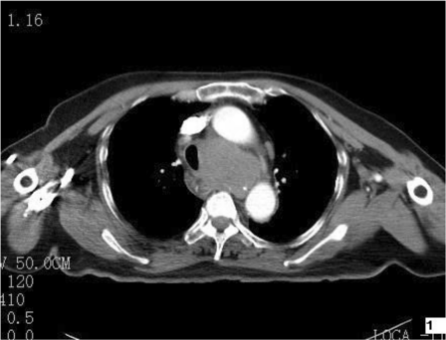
Axial contrast-enhanced CT image of the chest shows a well-defined lobulated homogeneous soft tissue mass in the superior middle mediastinum with peripheral speckle calcification and mild enhancement
